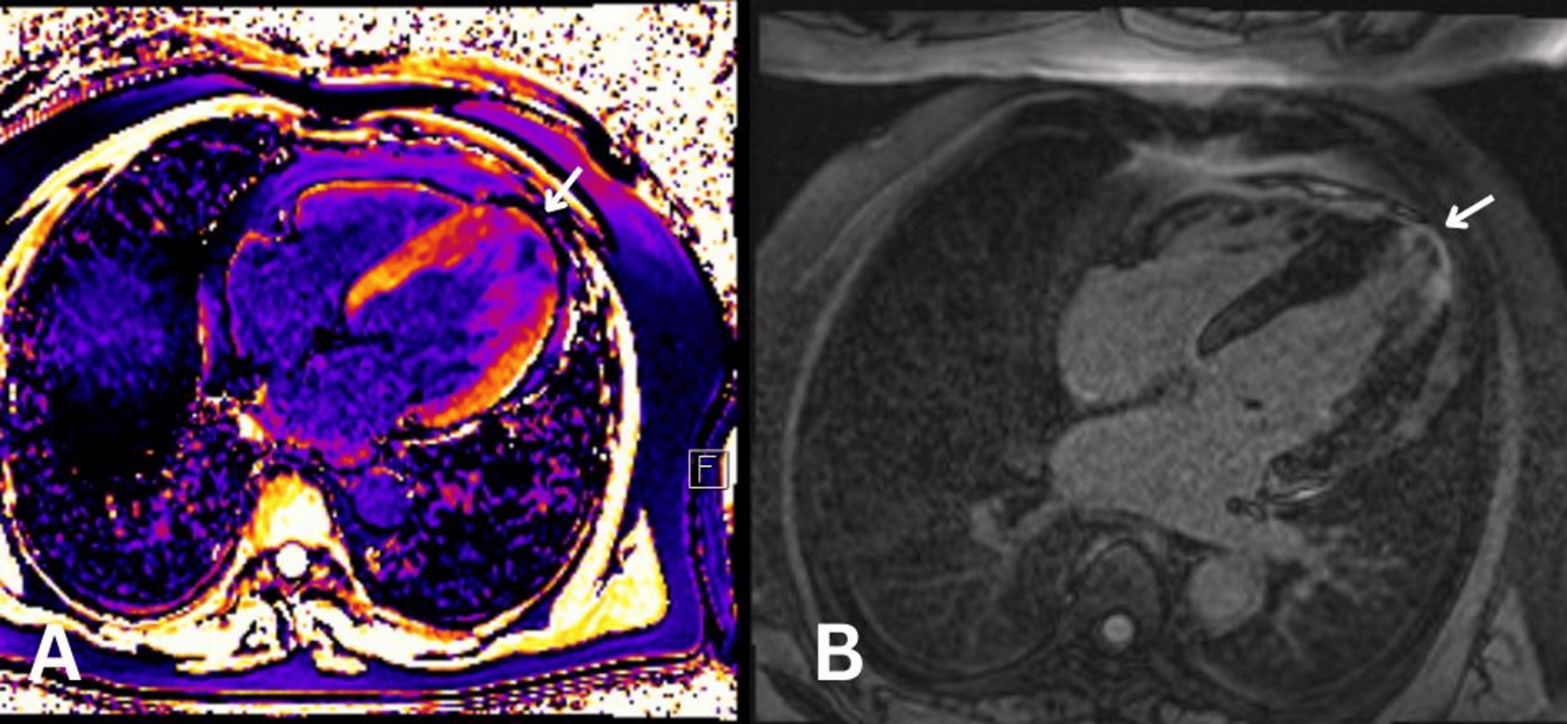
Cardiac MRI There is apical hypertrophy with noted A. T1 mapping showing an apical thrombus (the arrow points at the dark spot) and B. Delayed hyperenhancement showing a transmural post-ischaemic scar (the arrow points at the small ring of gadolinium accumulated in the expanded extracellular space). MRI: Magnetic resonance imaging
